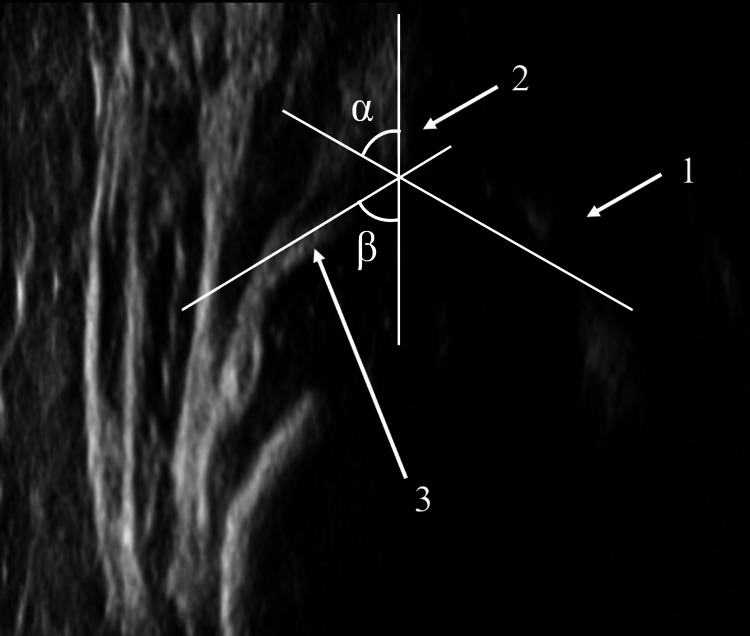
Sonographic findings of the infant hip joint Sonographic image of the infant hip on Graf’s standard plane. The alpha and beta angles of Graf's method are also shown in this figure. 1: Lower limb of the os ilium. 2: Mid-portion of the acetabular roof. 3: Acetabular labrum.
